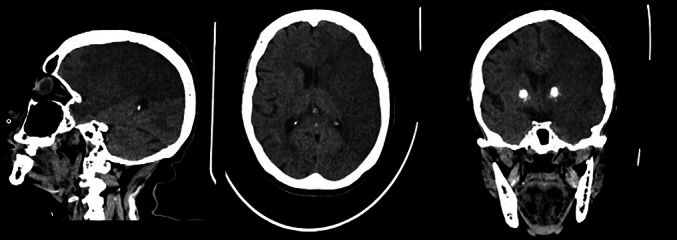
Head CT scan acute-subacute cerebral infarction in the cortical-subcortical area, prominent calcification of the right and left basal ganglia and calcification of the right and left vertebral arteries consistent with cerebral large vessel occlusion of the left middle cerebral artery.
